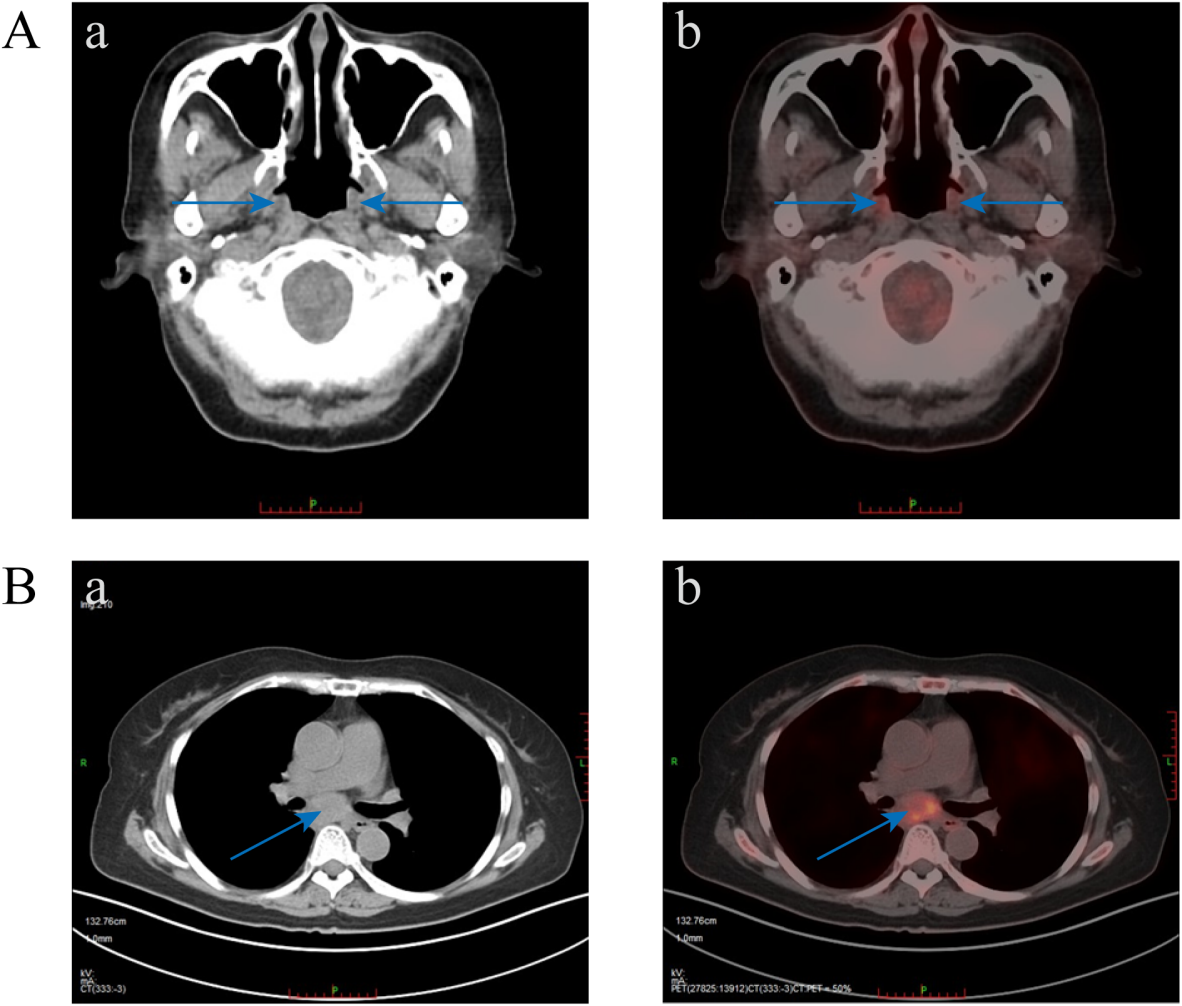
PET-CT images. (A) Diffuse mild increased metabolism of nasal alar, bilateral oral-buccal, bilateral periodontal, upper palate, oropharynx, laryngeal pharynx, and adjacent esophageal cervical mucosa was seen, which was considered to be caused by inflammation (blue arrow indicates increased metabolism area). (B) A mass of soft tissue in the mediastinum with increased metabolic heterogeneity.
